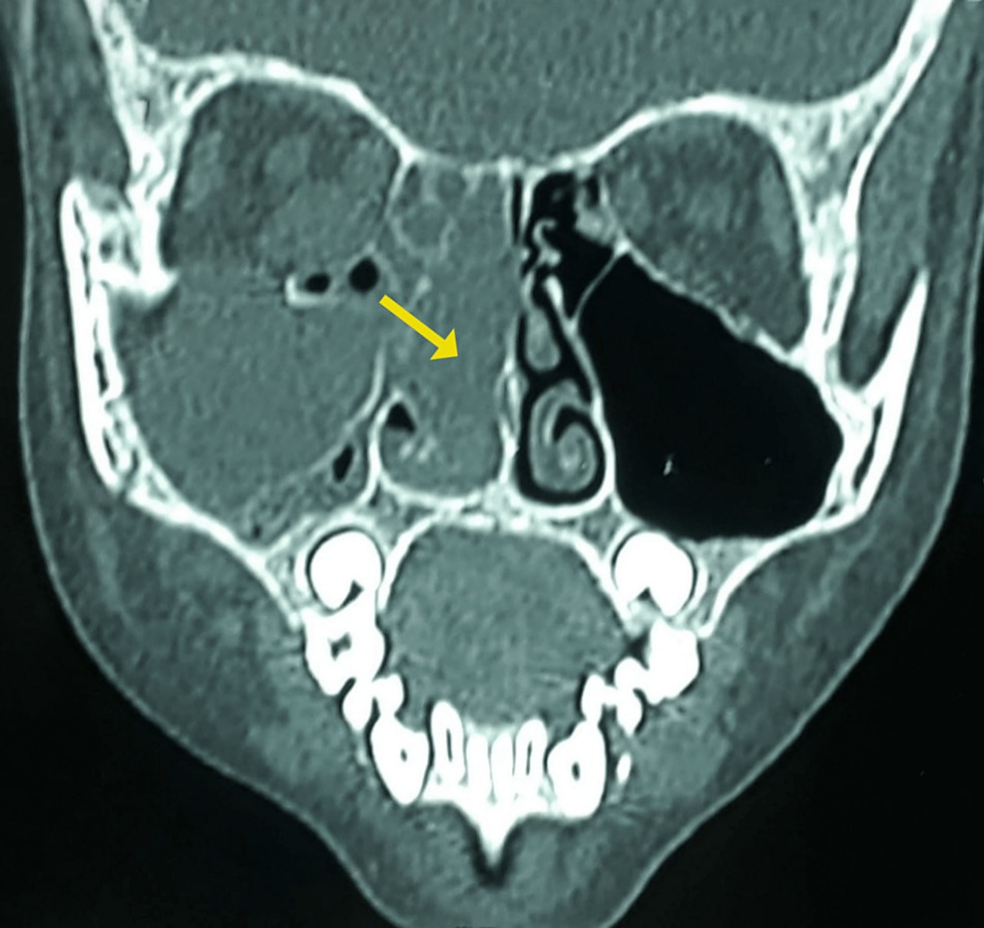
Coronal view showing soft density mass lesion occupying right nasal cavity and maxillary sinus as seen in computed tomography of paranasal sinuses
